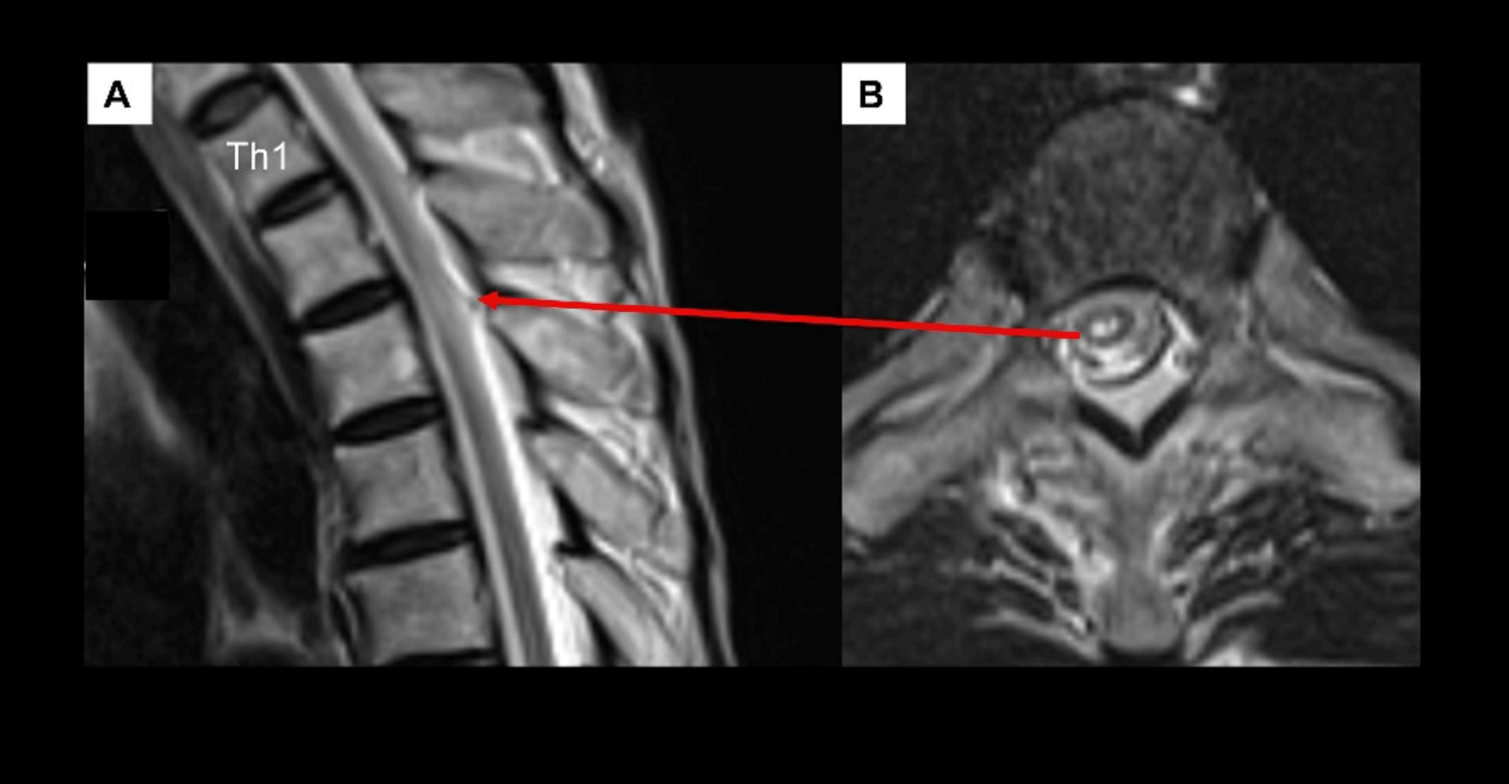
T2-weighted MRI of the thoracic spine after admission (A) Sagittal and (B) axial view of the T2-weighted MRI of the spine performed in mid-July demonstrated high signal intensity in the thoracic (Th2-Th3) region. There was no expansion of the lesion in the superior or inferior direction, and no edema or contrast effect was observed.
